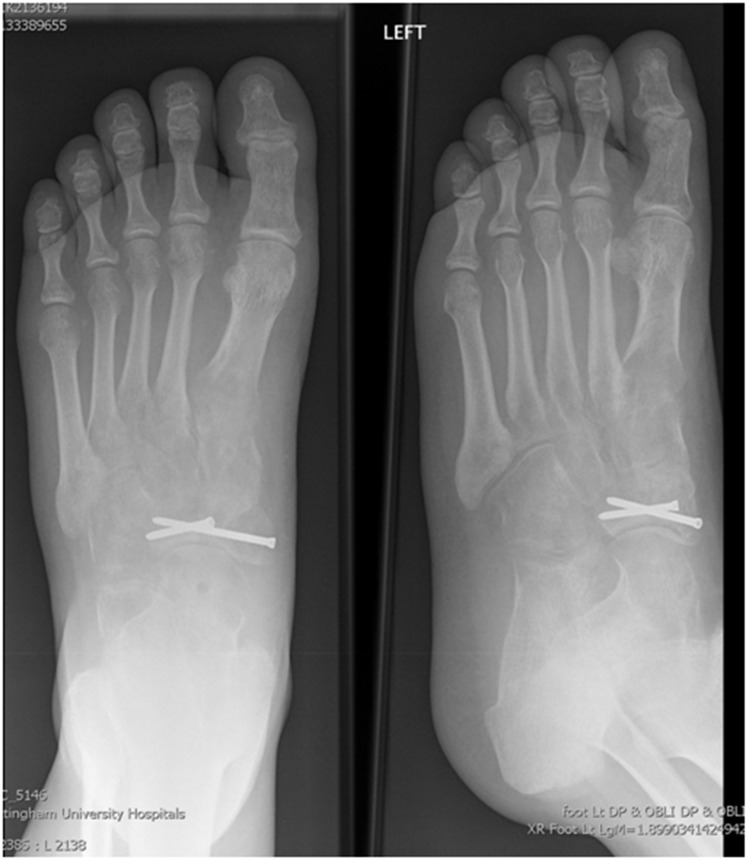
Weight bearing radiograph following removal of the bridge plate
